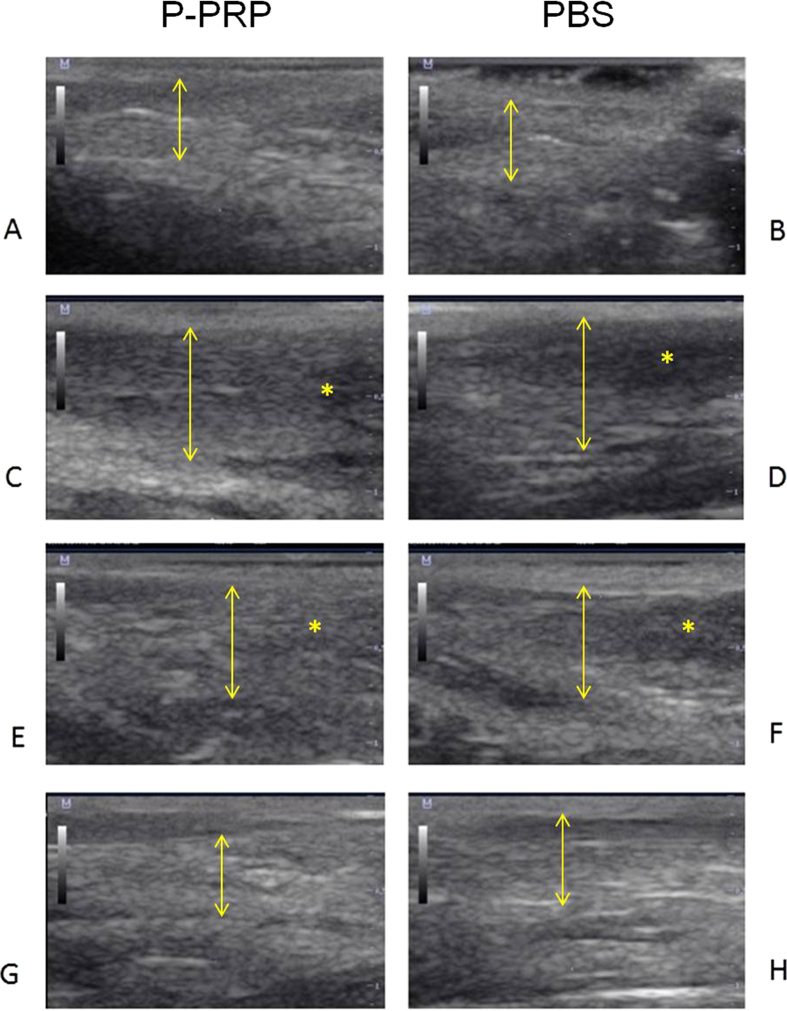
Tendon longitudinal ultrasonographic images. Normal basal tendon longitudinal ultrasonographic images in (A) LR-PRP- and (B) PBS-treated groups before collagenase tendinopathy induction. Tendon longitudinal ultrasonographic images in (C) LR-PRP- and (D) PBS-treated groups ten days after collagenase tendinopathy induction. Tendon longitudinal ultrasonographic images in (E) LR-PRP- and (F) PBS-treated groups 4 weeks after treatment. Tendon longitudinal ultrasonographic images in (G) LR-PRP- and (H) PBS-treated groups 12 weeks after treatment. ↕Tendon thickness indicator. Note that at any time no substantial differences are observed between both groups. However, there was an increase in the thickness of the tendons of both groups ten days after tendinopathy induction and 4 weeks after treatment. *Tendon damage indicator. Note hypoechoic zones in both groups ten days after tendinopathy induction and 4 weeks after treatment.
